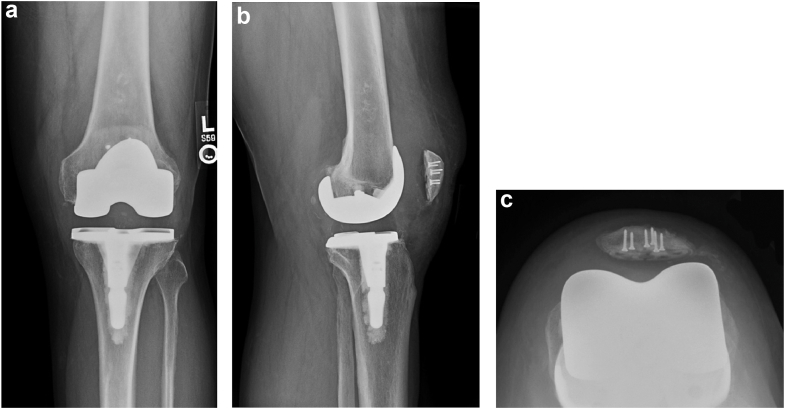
(a-c) Radiographs of revised patella 12 months postoperative. Anteroposterior radiograph (a) shows patella centrally overlying the trochlea. Lateral view (b) of patella demonstrates the cement-bone interface augmented with patellar rebar screws placed into the dorsal cortex. The sunrise view (c) suggests subtle residual lateral patellar tilt.
